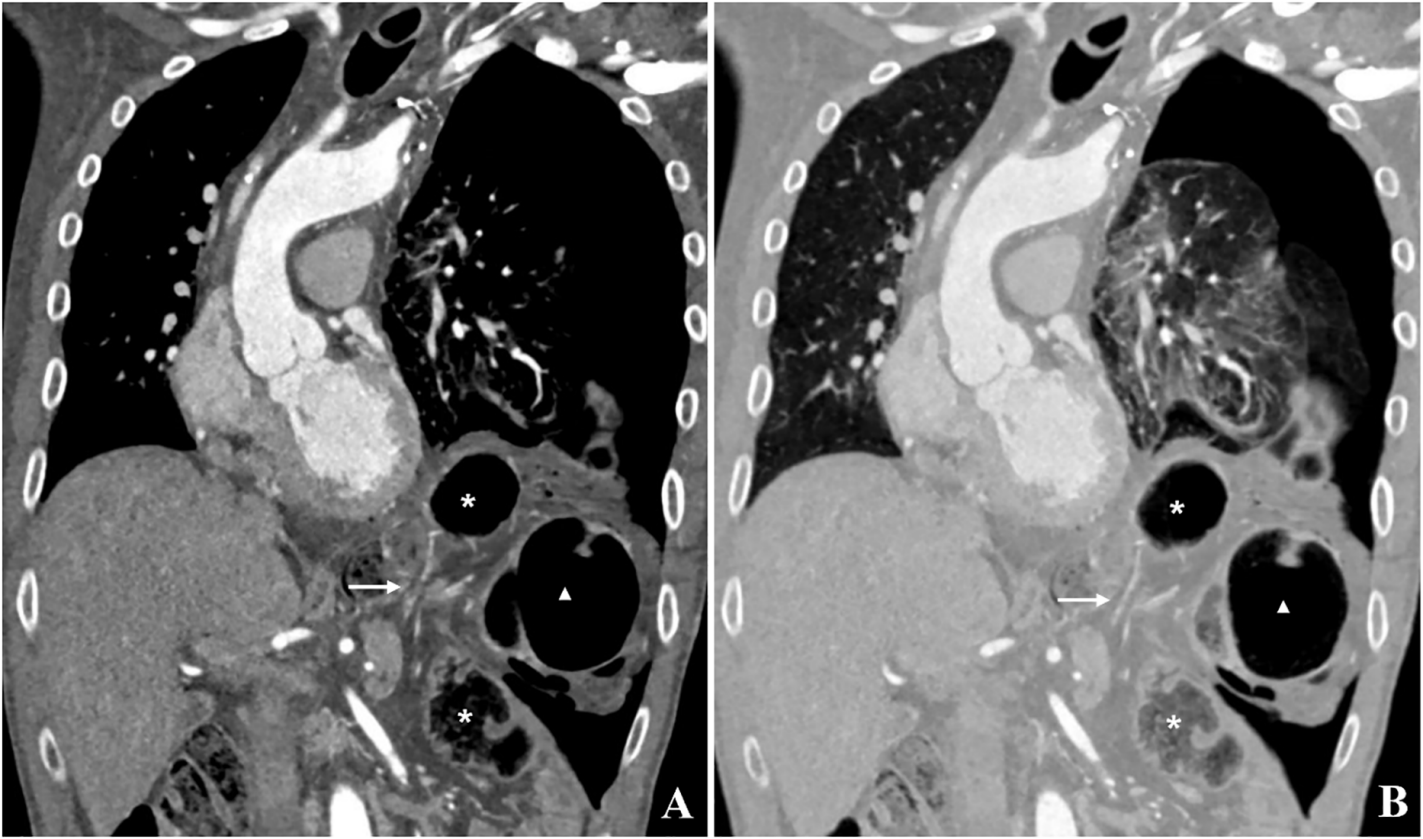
(A, B) CT image in coronal reconstruction. A diaphragmatic defect with twisted colon and mesocolon entering and exiting the left hemithorax can be seen. Due to twisting of the colon at the level of the diaphragmatic defect (Arrow) with subsequent closed-loop obstruction, the herniated intrathoracic colon loops are dilated (Star and Triangle). Arrow, Diaphragmatic defect; Star, Aboral part of the transverse colon; Triangle, Oral part of the transverse colon.
